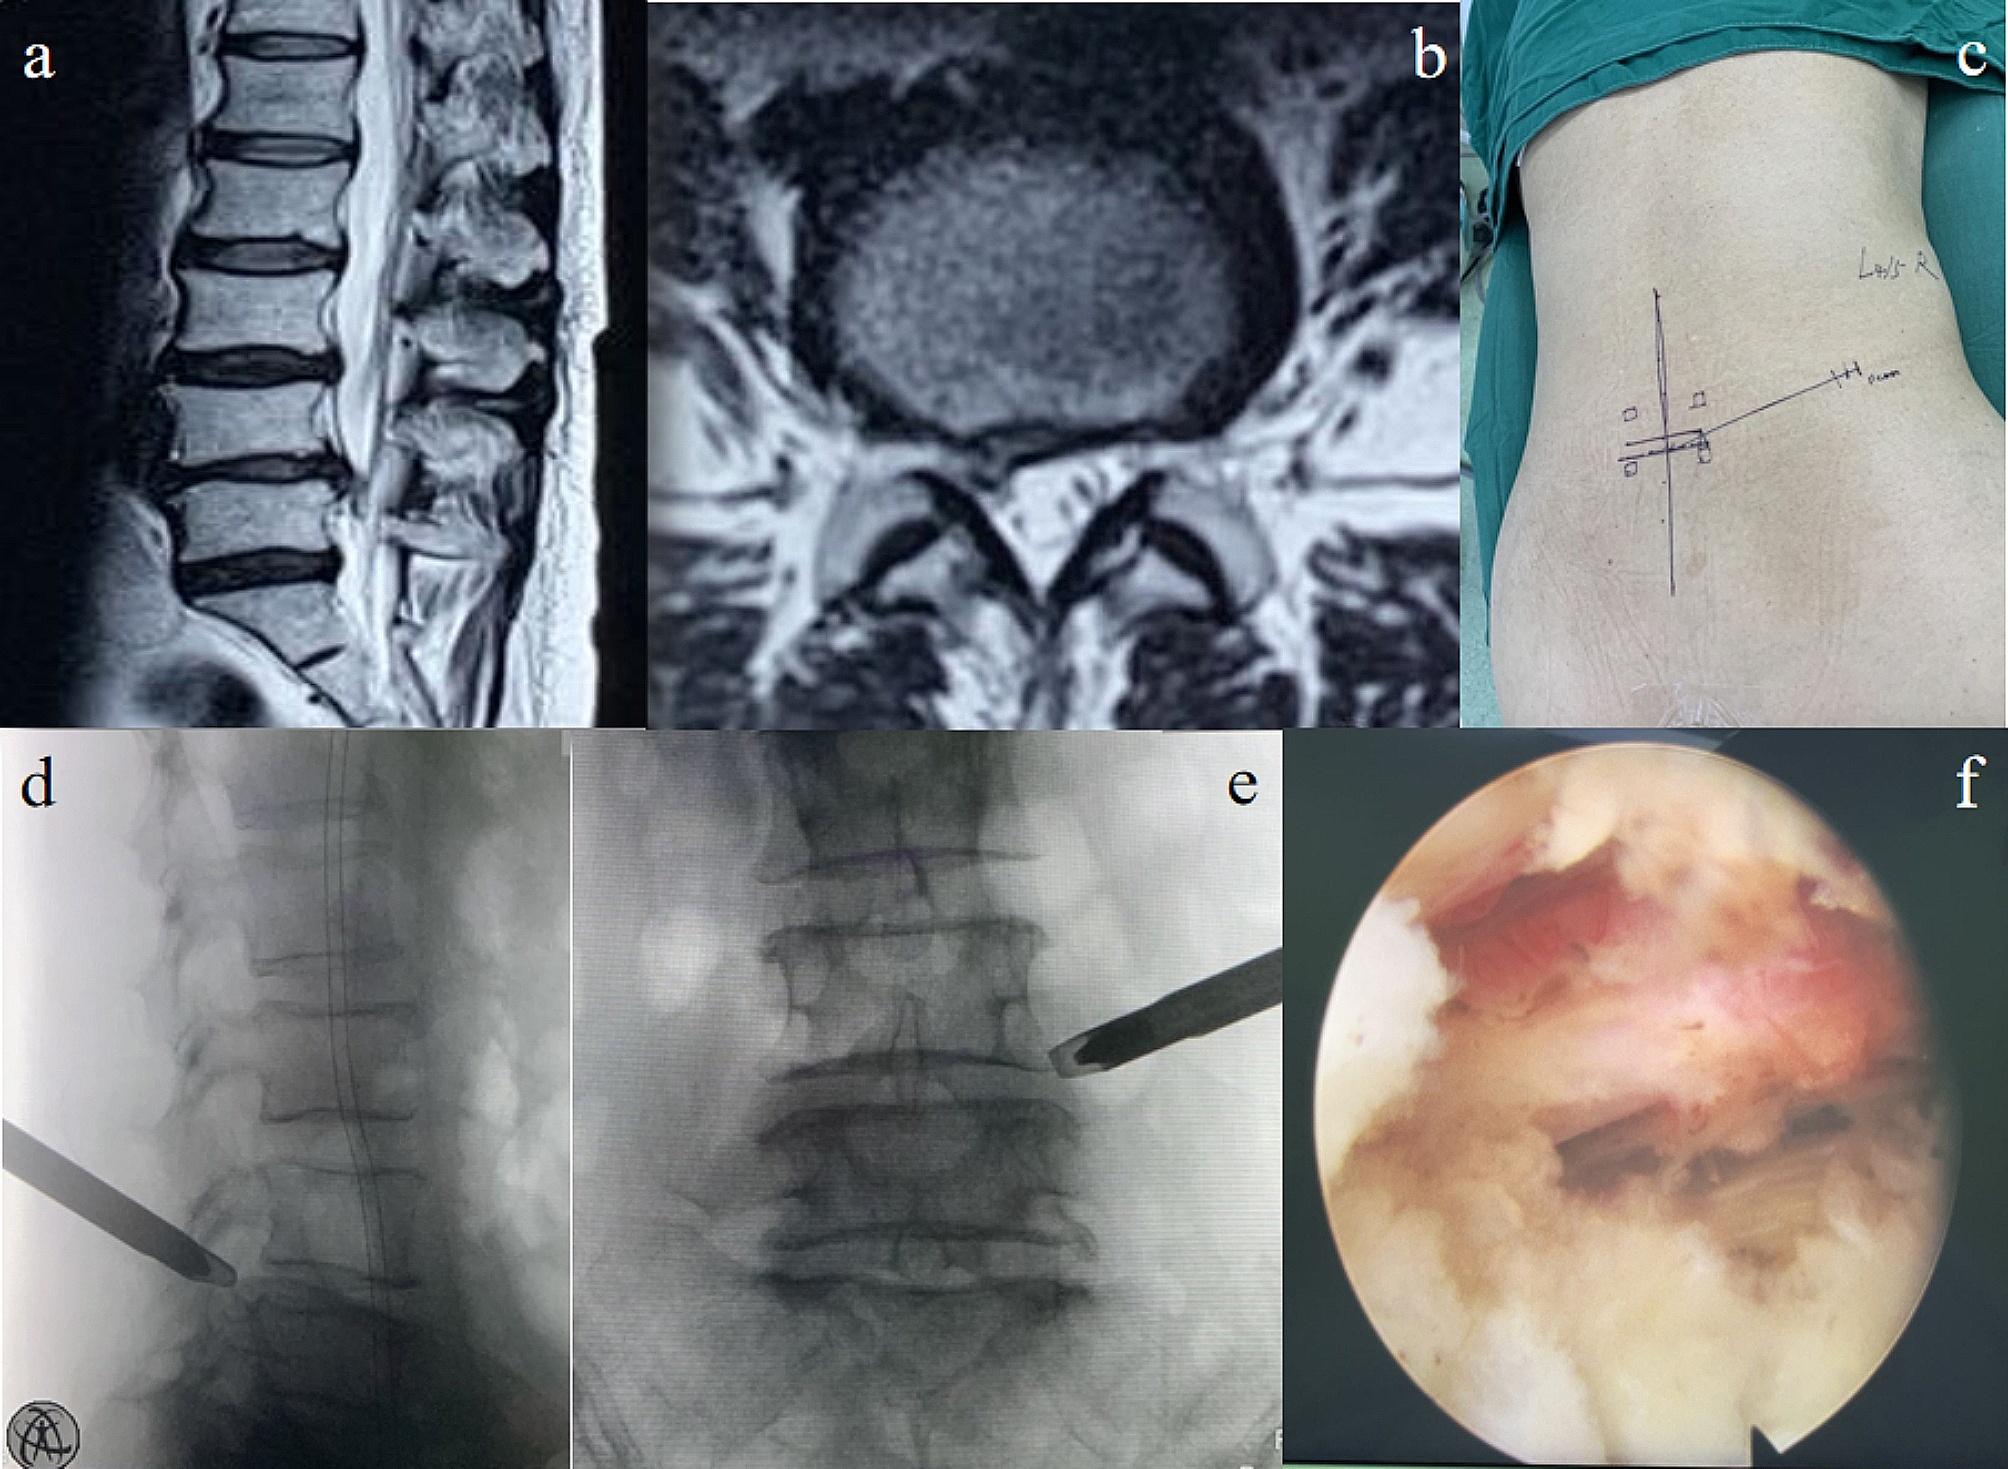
Images during treatment: (a, b) herniated intervertebral disc of L4/5 segment can be seen in the preoperative T2-weighted MRI; (c) surgical entry site markings; (d, e) Intraoperative fluoroscopy of the working channel of spinal endoscopy; (f) the view under the spinal endoscopy showed the ventral dura during the surgery
